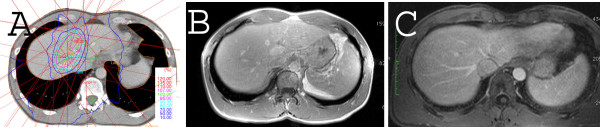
Radiation plan, pretreatment- and posttreatment MRI of a patient with two small liver metastases of a melanoma treated with 1x26Gy in 1 PTV. The lesions remain locally controlled (follow-up of 4 years).
